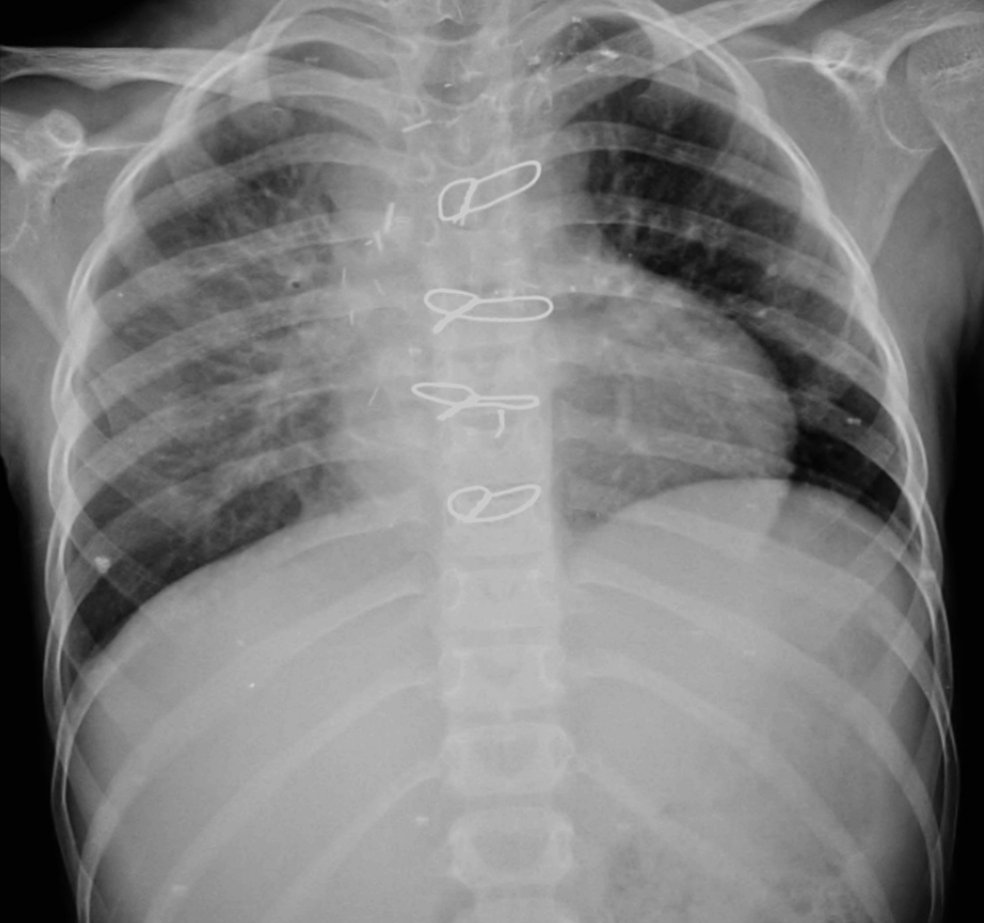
Chest X-ray post-embolization showing resolved bilateral pleural effusion
